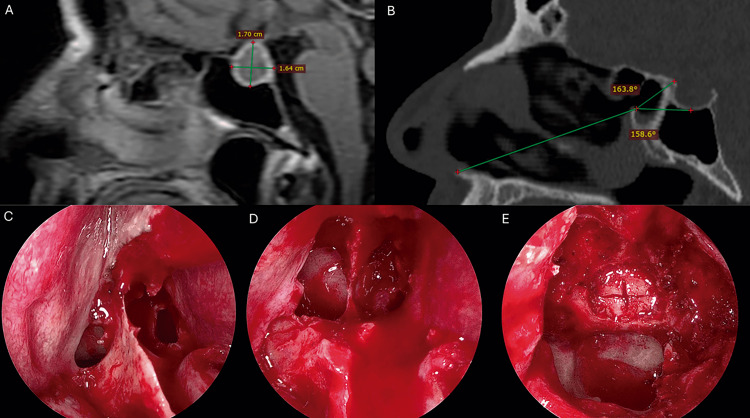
Non-functioning pituitary neuroendocrine tumor endoscopic endonasal transsphenoidal resection in a 72-year-old male A. Sagittal contrast enhanced magnetic resonance image displaying pituitary macroadenoma. B. Sagittal computed tomography exhibiting reduction of the Sellar and Tubercular angles. C. Sphenoidal rostrum exposition. D. Initial sphenoidotomy. E. Extended sphenoidotomy: posterior ethmoidectomy to expose tubercular recess, and rostrum removal to expose the sellar floor and clival recess, sellar floor drilling and cross-shaped aperture of the sellar dura is shown.
